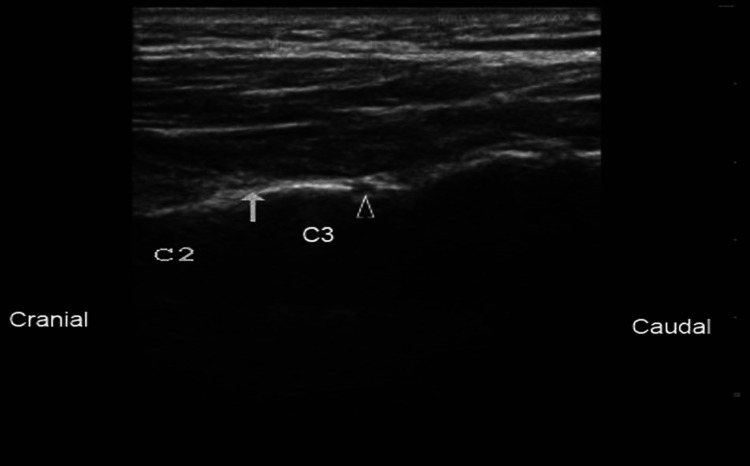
A view of the third occipital nerve obtained by ultrasound in the cervical spine from the same case as Figure 1. The arrows point to C2 and C3.
